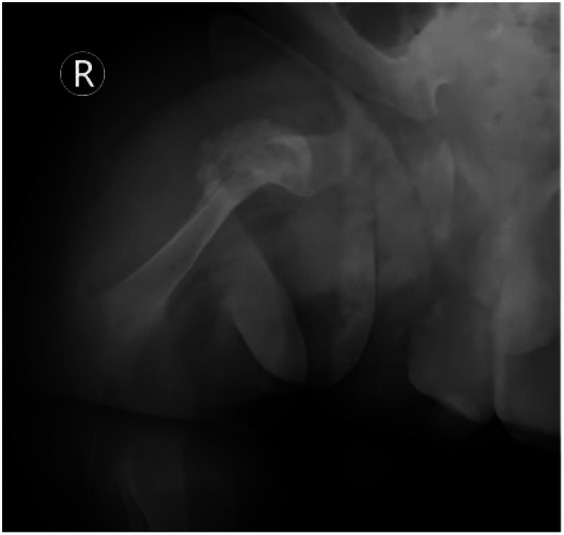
Radiographic evidence of rapid fracture healing (day 52 of life). Follow-up radiograph of the right femur reveals: satisfactory fracture alignment with a blurred fracture line and abundant cloud-like callus formation surrounding the site (red arrow). This image exemplifies the characteristic “rapid and hyperplastic” callus formation ability in OI, indicating successful initial healing and meeting the criteria for harness removal.
